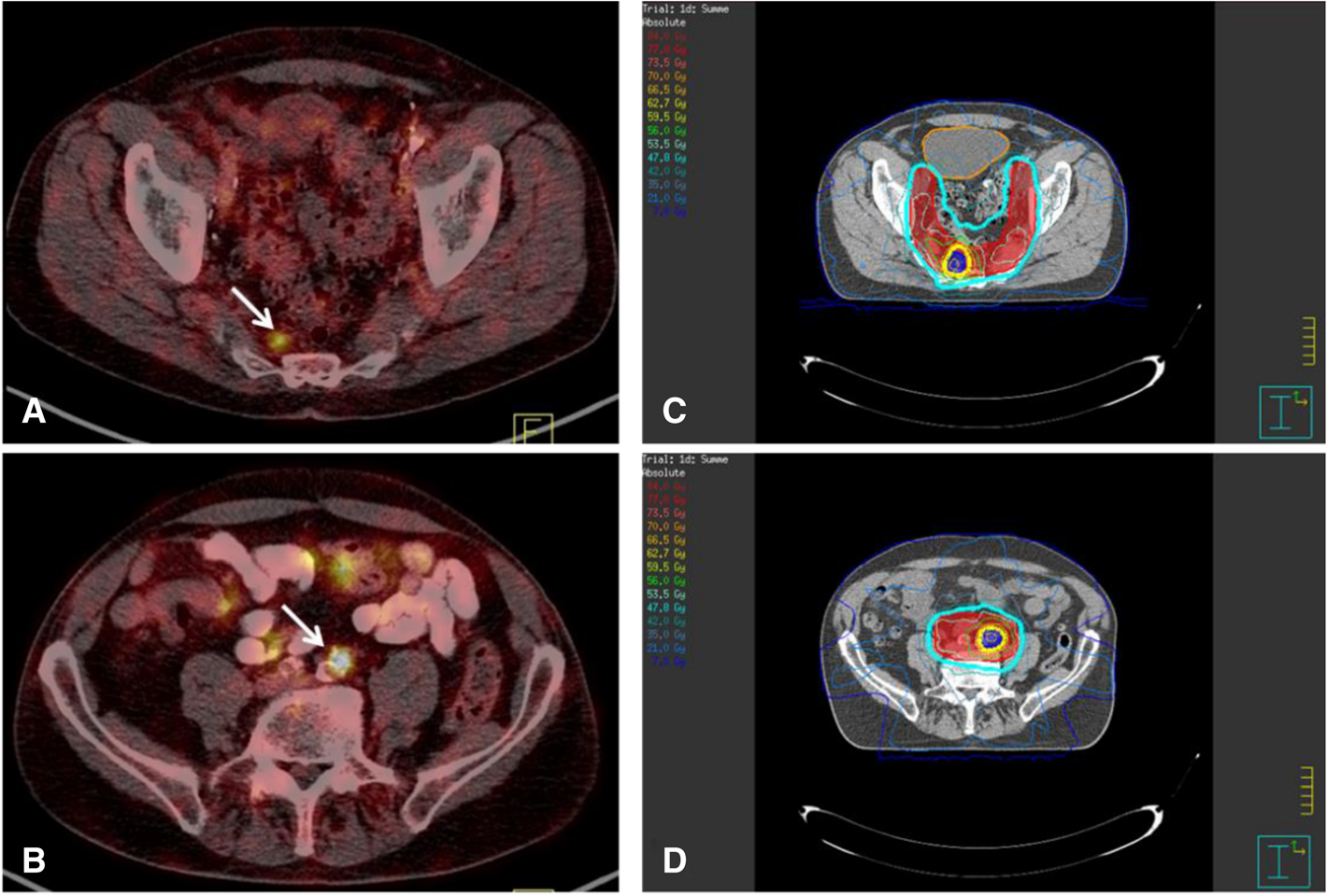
A 74-year-old patient with biochemical recurrence (PSA 0.82 ng/ml; pT2aN0cM0; Gleason 6; iPSA 5.37 ng/ml) 8.4 months after radical prostatectomy and lymph node dissection. ^68^Ga-PSMA PET/CT prior to salvage radiotherapy showed two PSMA-positive presacral (a, c) and retroperitoneal (b, d) LNMs. Salvage radiotherapy was extended to pelvic lymph nodes, including a dose escalation to the PSMA-positive lymph nodes. The patient was treated with IMRT (c, d IMRT plan). *red* PTV including pelvic lymph nodes (50.4 Gy), *blue* simultaneous and sequential boost (66 Gy) for iliac (d) and presacral (c) LNM. RT to prostate bed is not shown. The PSA level decreased to 0.02 ng/ml after SRT
